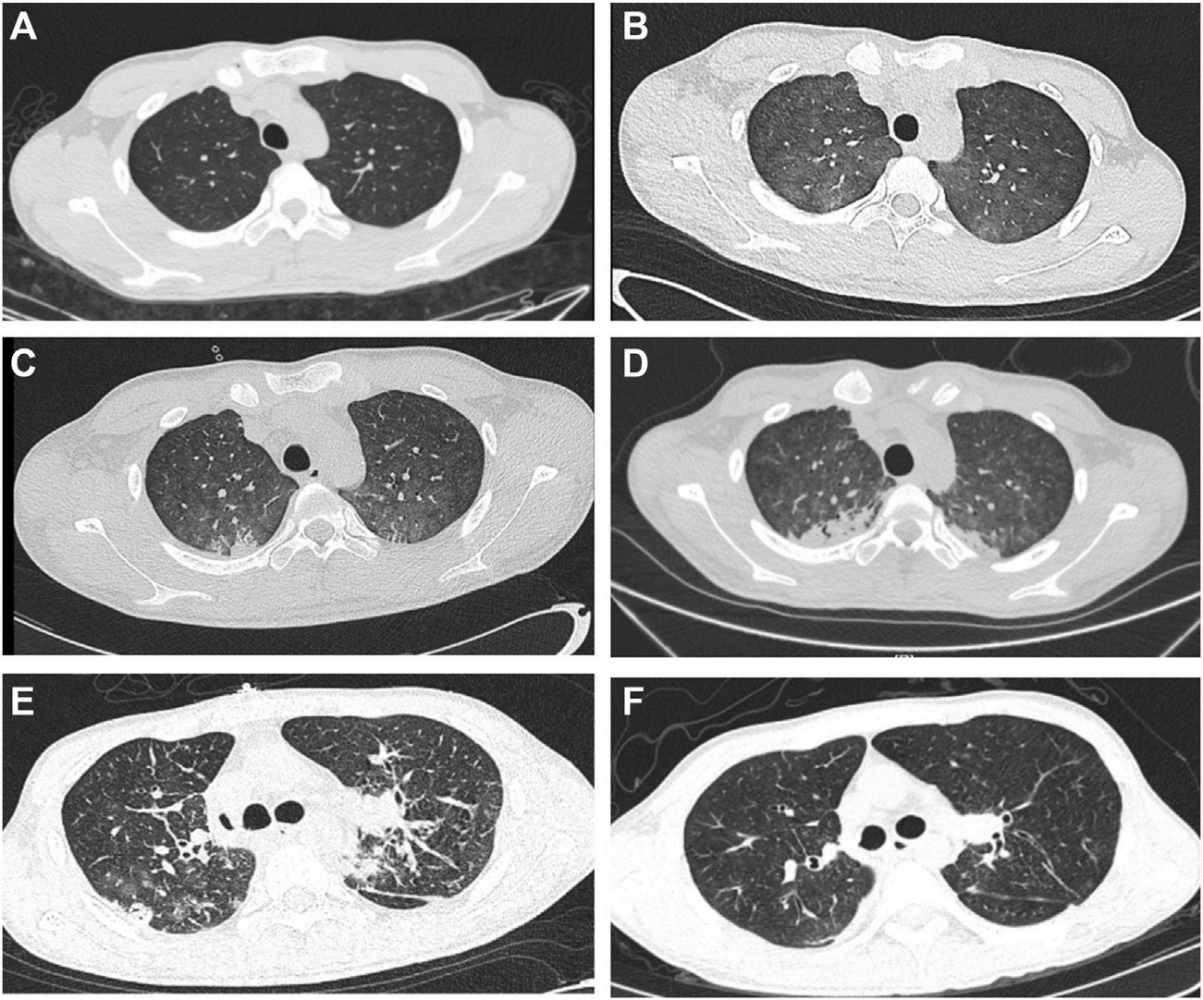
Preoperative CT images and postoperative CT images (A–D) Progression of bilateral lung fibrosis. (E, F) Post-lung transplantation CT shows normality and without fibrosis.
